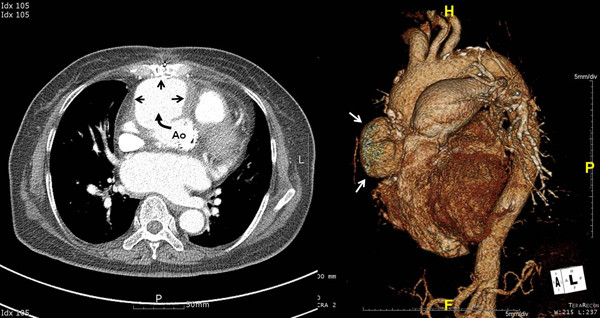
The preoperative chest CT scan shows a large pseudoaneurysm in the ascending aorta (black and white arrows).
